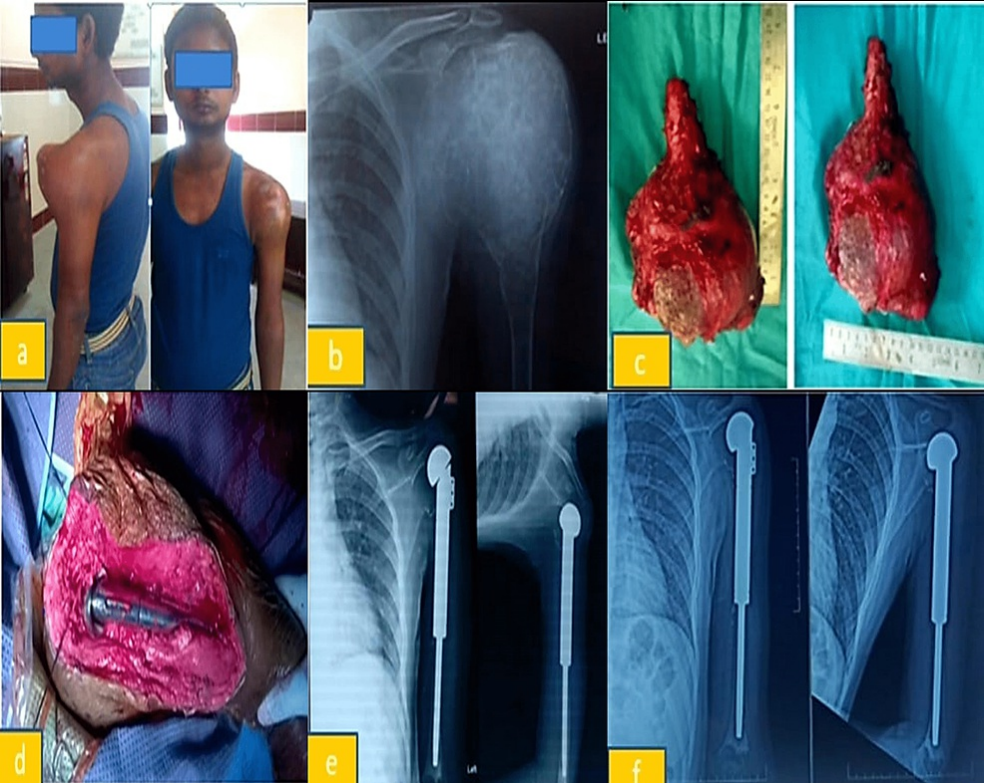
Chondromyxoid fibroma of the left proximal humerus. (a) Pre-operative photograph of a patient with chondromyxoid fibroma of the left proximal humerus. (b) Pre-operative x-ray of the left proximal humerus. (c) Excised left proximal humerus tumour. (d) Intraoperative photograph of the implanted left proximal humerus mega prosthesis. (e) Three-month post-operative x-ray. (f) Six-year x-ray follow-up.
